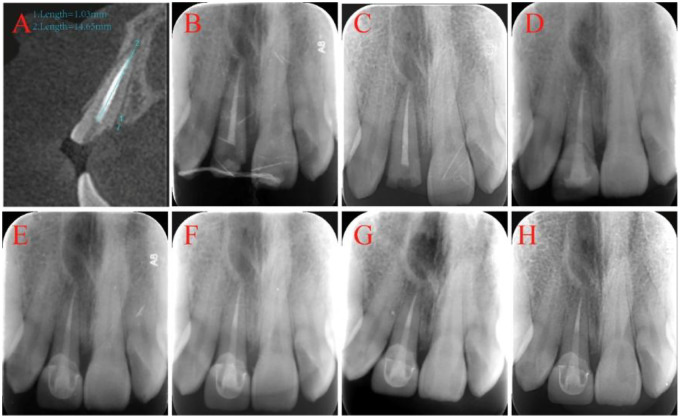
CBCT and radiographs image: (A) CBCT image after the second trauma; (B) post-intentional replantation; (C) 7-day follow-up; (D) 6-month follow-up; (E) 1-year follow-up; (F) 2-year follow-up; (G) 3-year follow-up; (H) 4-year follow-up.
